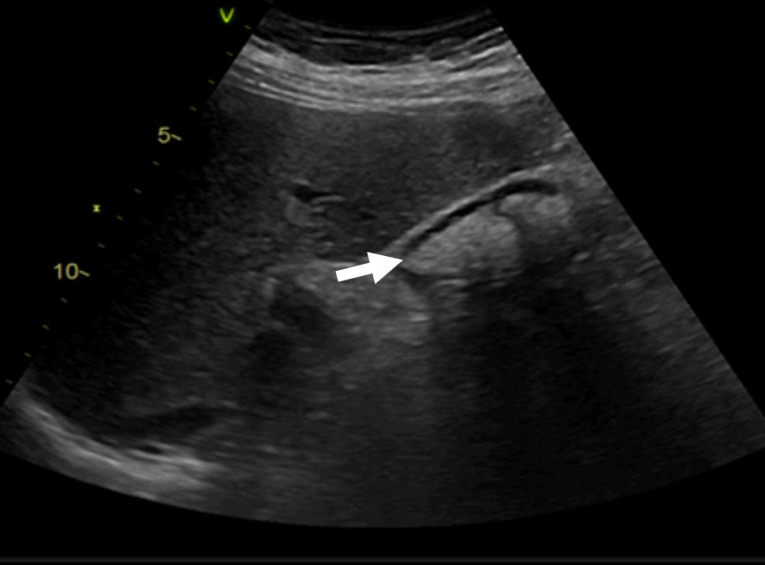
Abdominal ultrasound showing biliary sludge (arrow) associated with ceftriaxone therapy.
